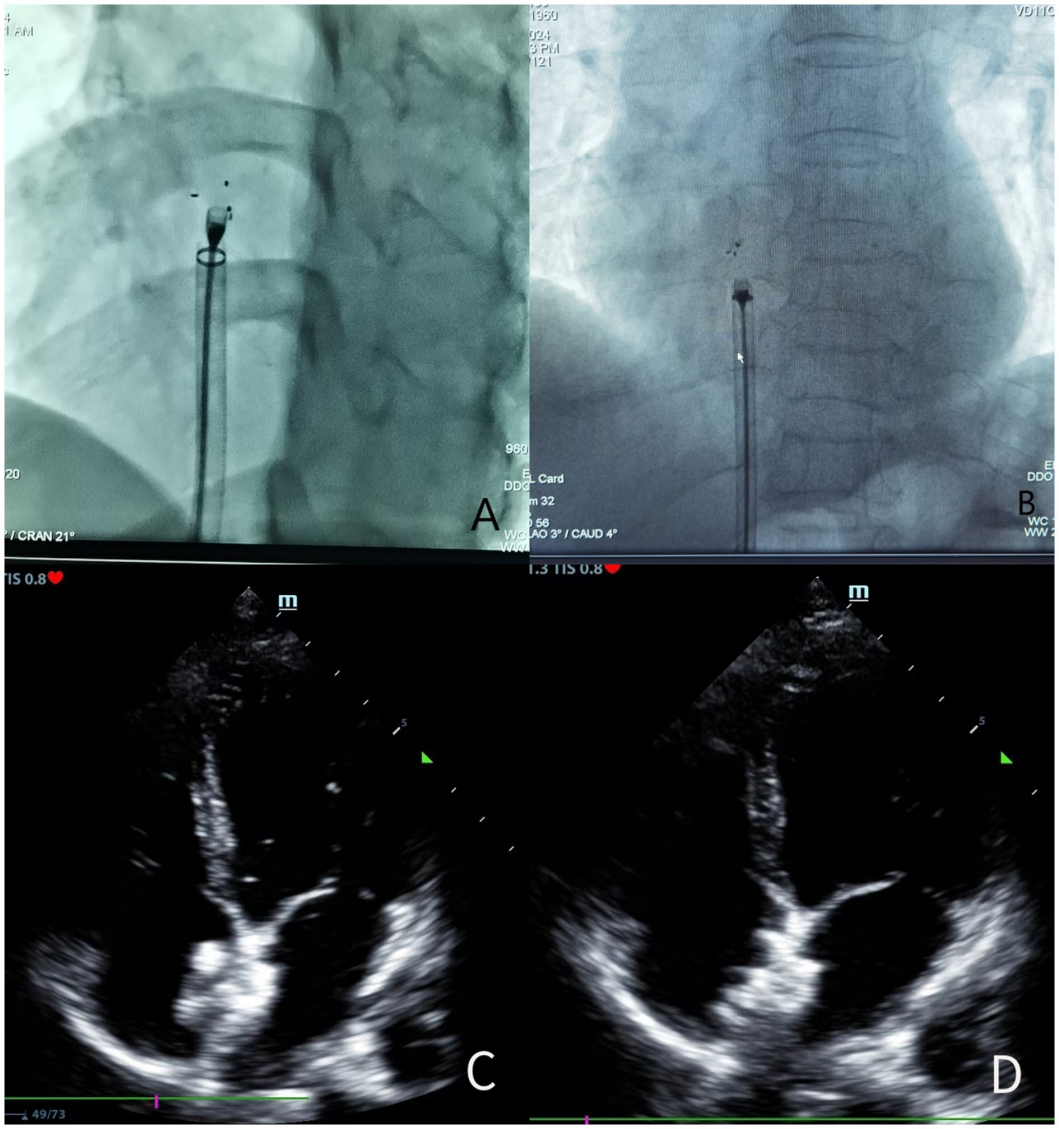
(A) Before the release of the biodegradable occluder under the X-ray line; (B) After the release of the biodegradable occluder under the X-ray line; (C) Before the release of the biodegradable occluder by TTE during the operation; (D) After the release of the biodegradable occluder by TTE during the operation.
